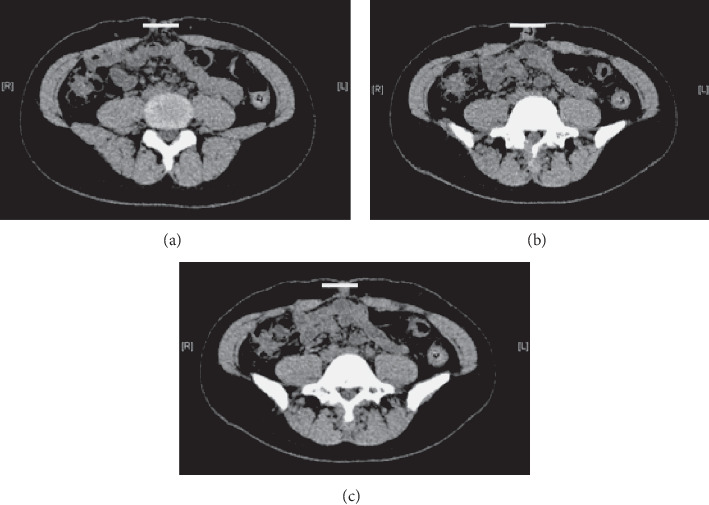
Preoperative abdominal computed tomography: (a) 3 cm above the superior border of the umbilicus; (b) center of the umbilicus; (c) 2 cm below the inferior border of the umbilicus. The inter-rectus distance is indicated by a white line.
